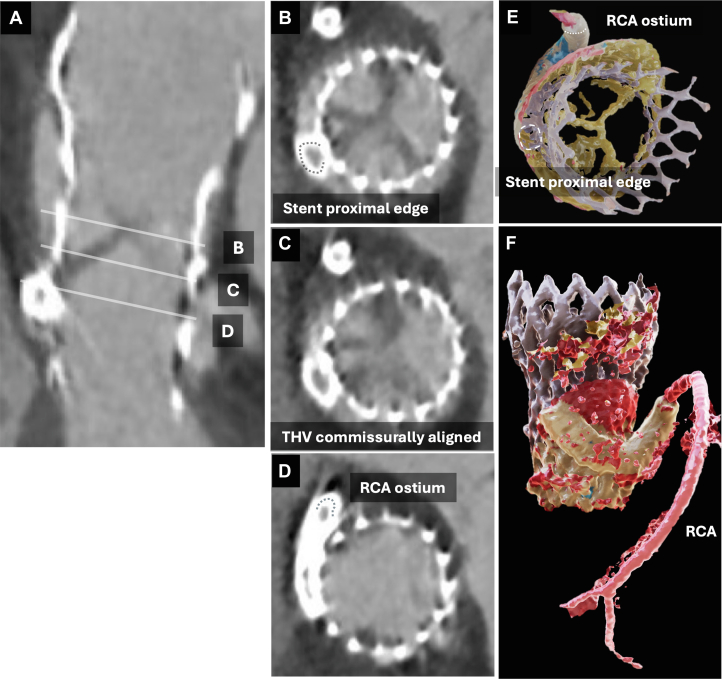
Computed Tomography and 3-Dimensional Reconstruction Images at 6-Month Follow-Up (A to D) At 6 months, the stents were positioned at the proximal RCA via a route from the NCS through the transcatheter heart valve strut above the RCS. (B to E) Detailed computed tomography assessment demonstrates that commissural alignment was successfully achieved and considered favorable. (E and F) The roundabout approach is clearly visualized through the 3-dimensionally reconstructed images (reconstructed using Viewtify, Sciement). Abbreviations as in Figure 4.
